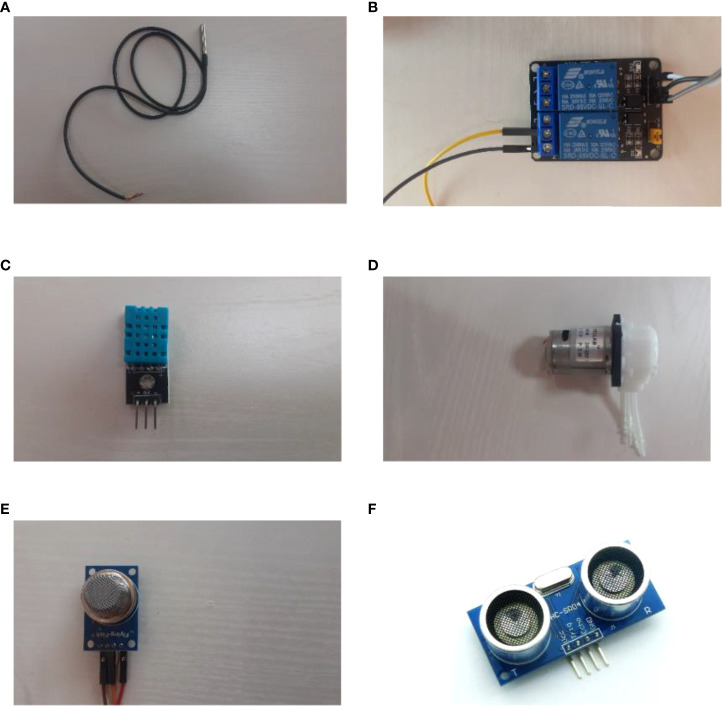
(A) DS18B20 sensor, (B) Two channel DC 5V relay module, (C) DHT-11 temperature and humidity sensor, (D) peristaltic pump, (E) MQ135 CO2 sensor, (F) ultrasonic sensor.
